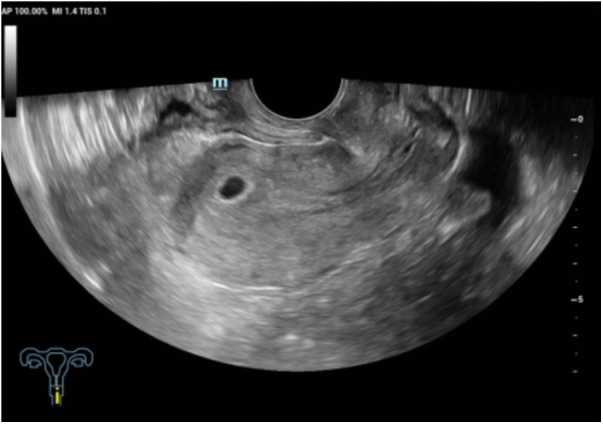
Five days after the operation, transvaginal color Doppler ultrasound showed the echo of the gestational sac in the uterine cavity, and the size was about 10 × 6 × 7 mm.
